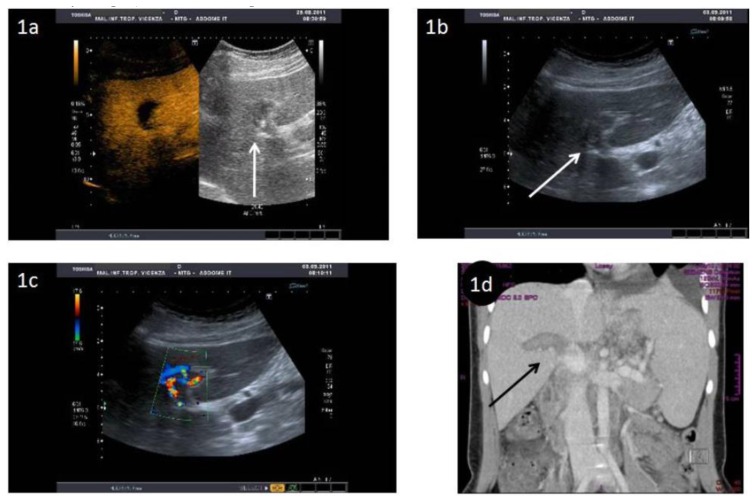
1a- Patient One: CEUS and US on admission, showing the thrombus in the left portal branch (arrow). 1b- Patient One: US on discharge, showing the thrombus organization (retraction and hyperechoic clot) (arrow). 1c- Patient One: US-Doppler on discharge, showing partial revascularization. 1d- Patient Two: coronal scan of abdominal contrast media CT. The hypodense material is inside the portal vein (arrow) and the spleen is enlarged.
